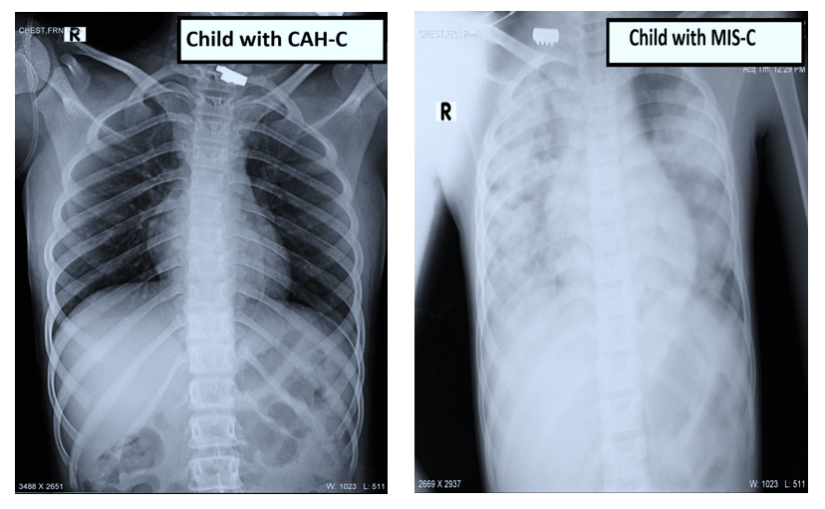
The x-ray findings of a child with CAH-C versus a child with MIS-C. CAH-C: COVID-19–associated hepatitis in children; MIS-C: multisystem inflammatory syndrome in children.
